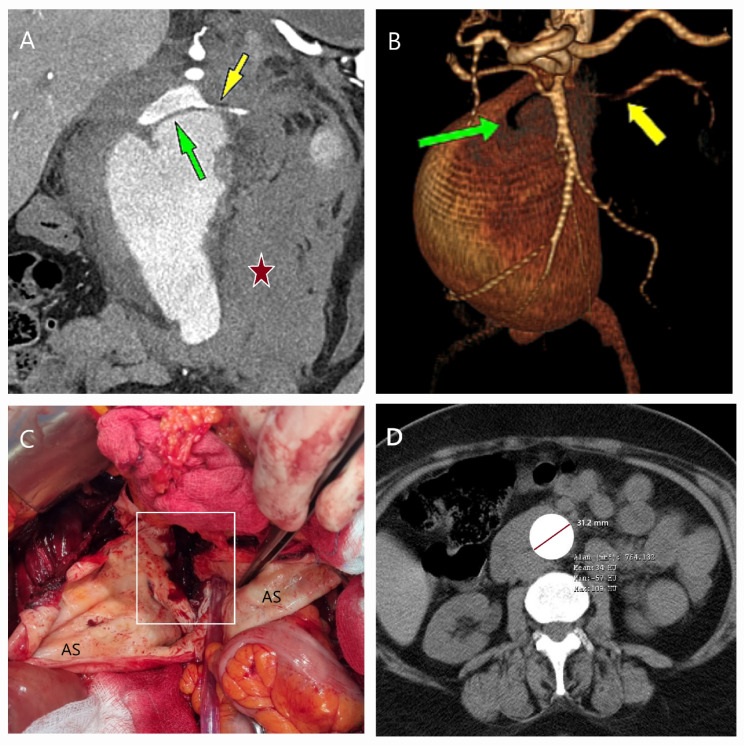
Silent aneurysm growth over six years: CT and surgical images. (A,B) A 57-year-old female smoker, undergoing hypertension treatment and socially active, presented with severe abdominal pain and syncope. Her poor clinical condition, absence of an aneurysm neck, dissection (green arrow) extending from the left renal artery (yellow arrow), and the presence of a hematoma (red star) necessitated open surgery. (C) Intraoperatively, a 7 cm longitudinal rupture (white square) was identified on the posterior aneurysm wall (AS, Aneurysm sac wall). (D) Retrospective review of a CT scan from six years earlier, initially performed for urinary stone evaluation, revealed an abdominal aorta measuring only 3 cm. (Alan; which means ‘area’ in English). Unaware of its silent progression, the patient lived for six years without intervention. This case underscores the importance of early detection of aortic pathology across all clinical disciplines and reinforces the need for population-based screening programs.
